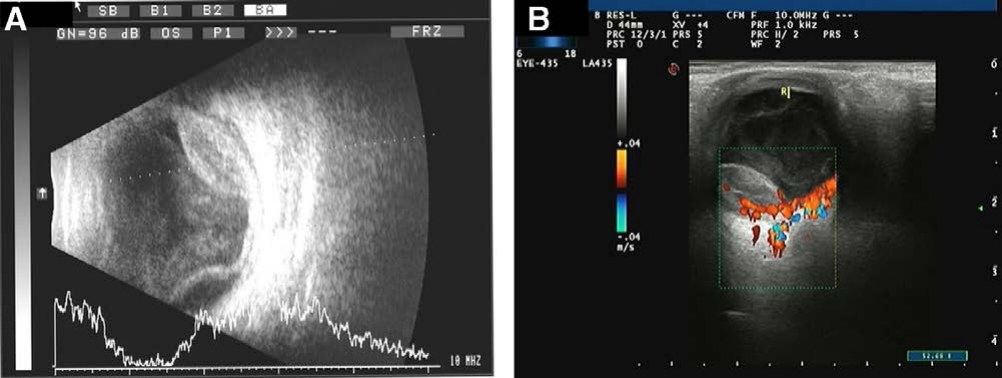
B-scan (A) and color ultrasound (B) revealed a dome-shaped subretinal mass with exudative retinal detachment.
